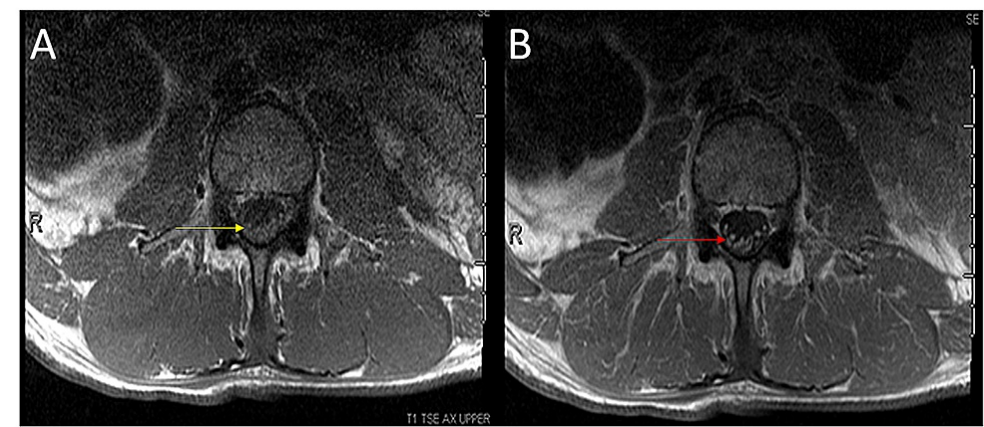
MRI lumbar spine with and without gadolinium A. MRI lumbar spine T1 axial image with unenhanced nerve roots (yellow arrow). B. MRI lumbar spine T1 post-gadolinium axial image with the enhancement of ventral nerve of the cauda equina (red arrow) MRI: magnetic resonance imaging
